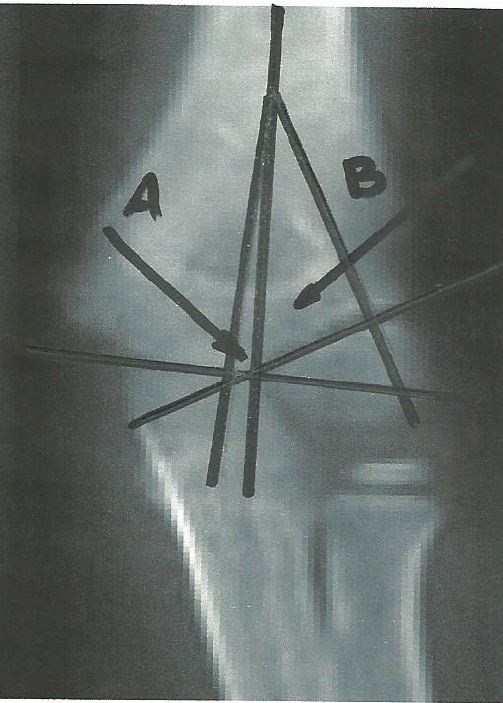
Follow-up radiographs 2 years after fracture of a cubitus valgus elbow. In this case the TCI is less than 0.18.
